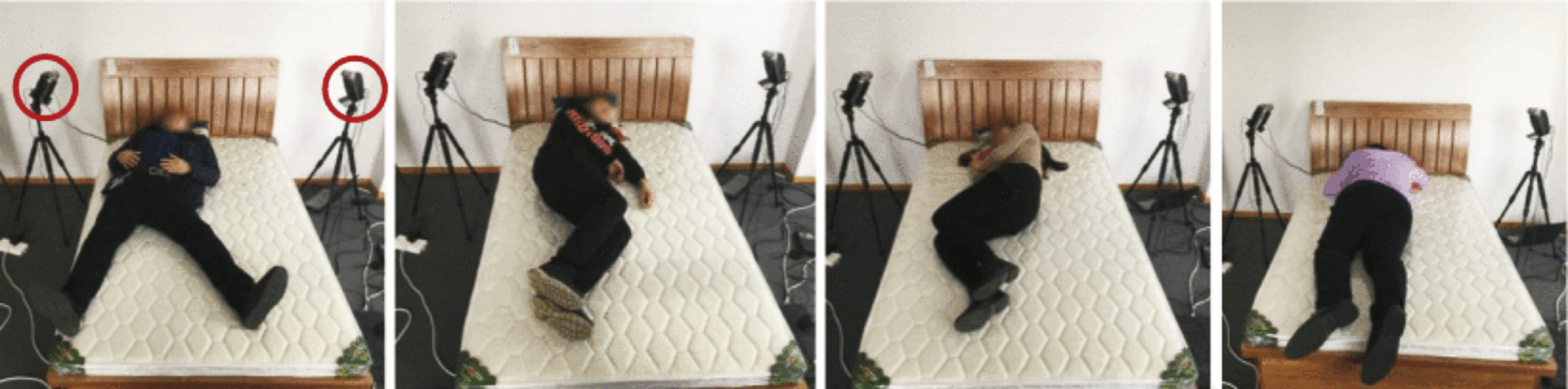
Experimental setup to monitor abnormal respiratory using ultrasound signals (reprinted from Ref. [99] with permission).
